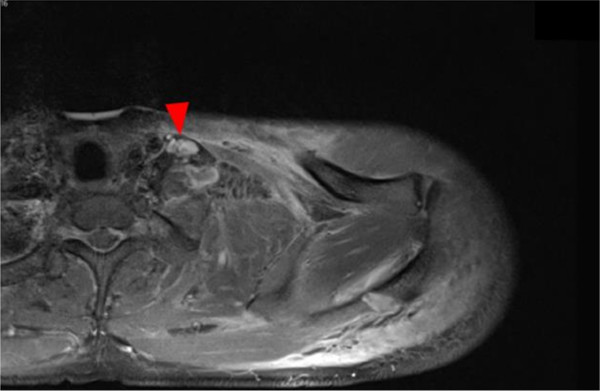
MRI of left shoulder girdle. Tear of the left brachial plexus.
